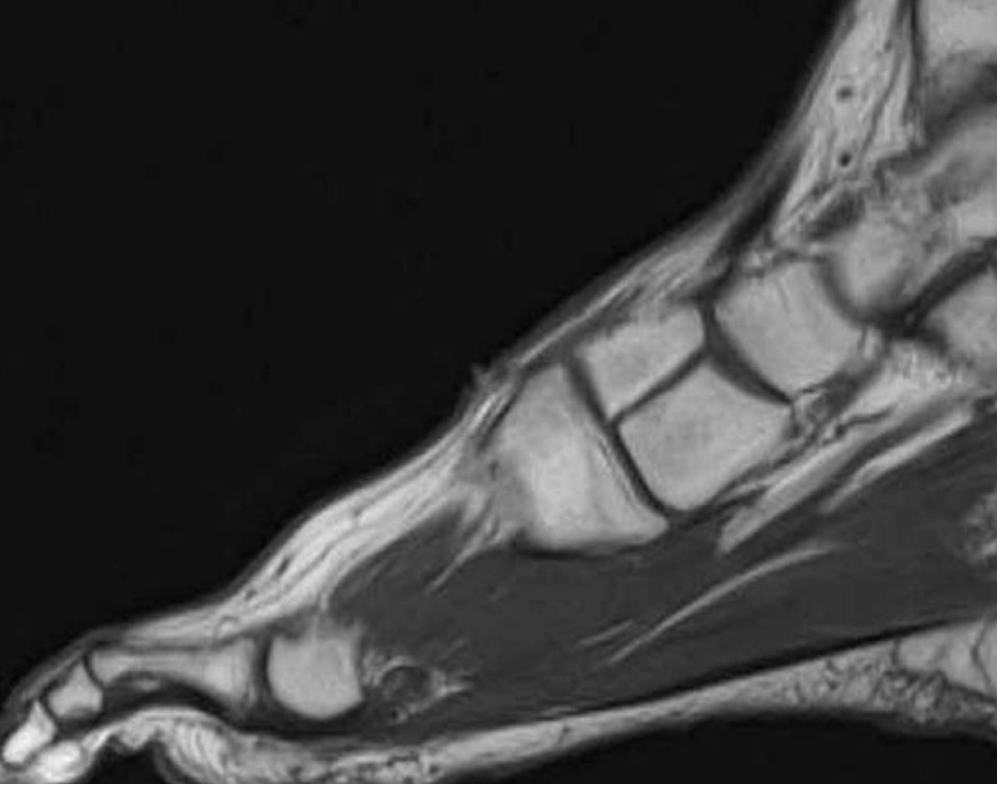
Sagittal T1 weighted spin echo MR image (TR/TE = 500/12 ms) demonstrates a typical bipartite medial cuneiform noted in case #1.
